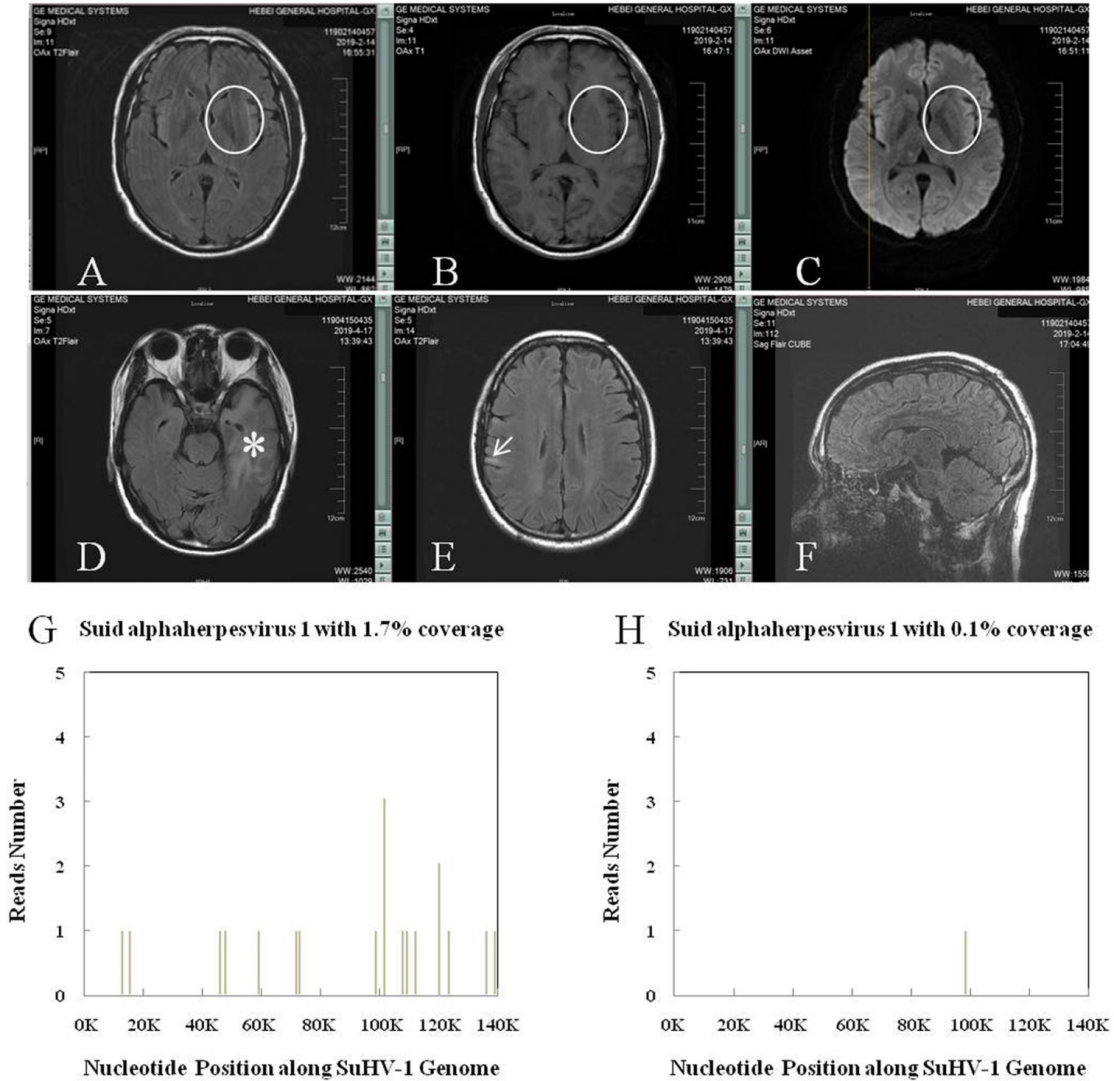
SuHV-1 encephalitis in patient of chronic kidney disease stage 4 and the high throughput sequencing results. A, B and C: Brain MRI showed intense T2 Flair, T1 and DWI signal changes in the left basal ganglia (circle). D and E: Brain MRI showed intense T2 Flair signal changes in the left hippocampus and temporal lobe (asterisk), and right frontoparietal lobe (arrow), respectively. F: CUBE technique found no sign of meningitis. G: Next-generation sequencing of the cerebrospinal fluid detected SuHV-1 virus with 16 unique sequence reads and 1.7% coverage. H: Next-generation sequencing of the cerebrospinal fluid detected SuHV-1 virus with 1 unique sequence reads and 0.1% coverage 22 days after treatment.
